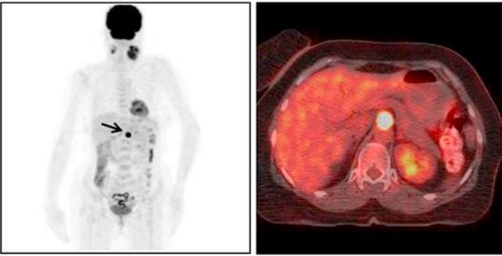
FDG-Positron Emission Tomography (PET) CT scans showed a mass (SUVmax: 28.3) detected at the celiac truncus level in front of the abdominal aorta (arrow).
